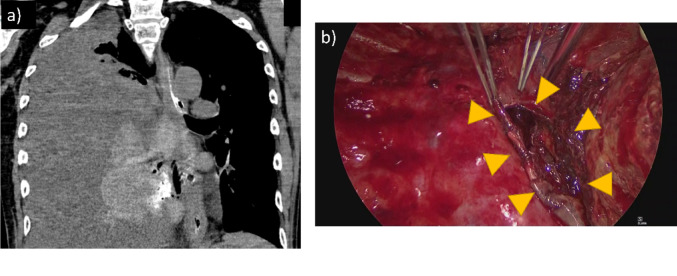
a Chest CT on day 4 after admission showing extravasation of contrast medium from the mid-thoracic esophagus into the right pleural cavity. b Intraoperative findings showing a 4-cm longitudinal tear of the esophageal muscular layer in the mid-thoracic esophagus (yellow arrows)
